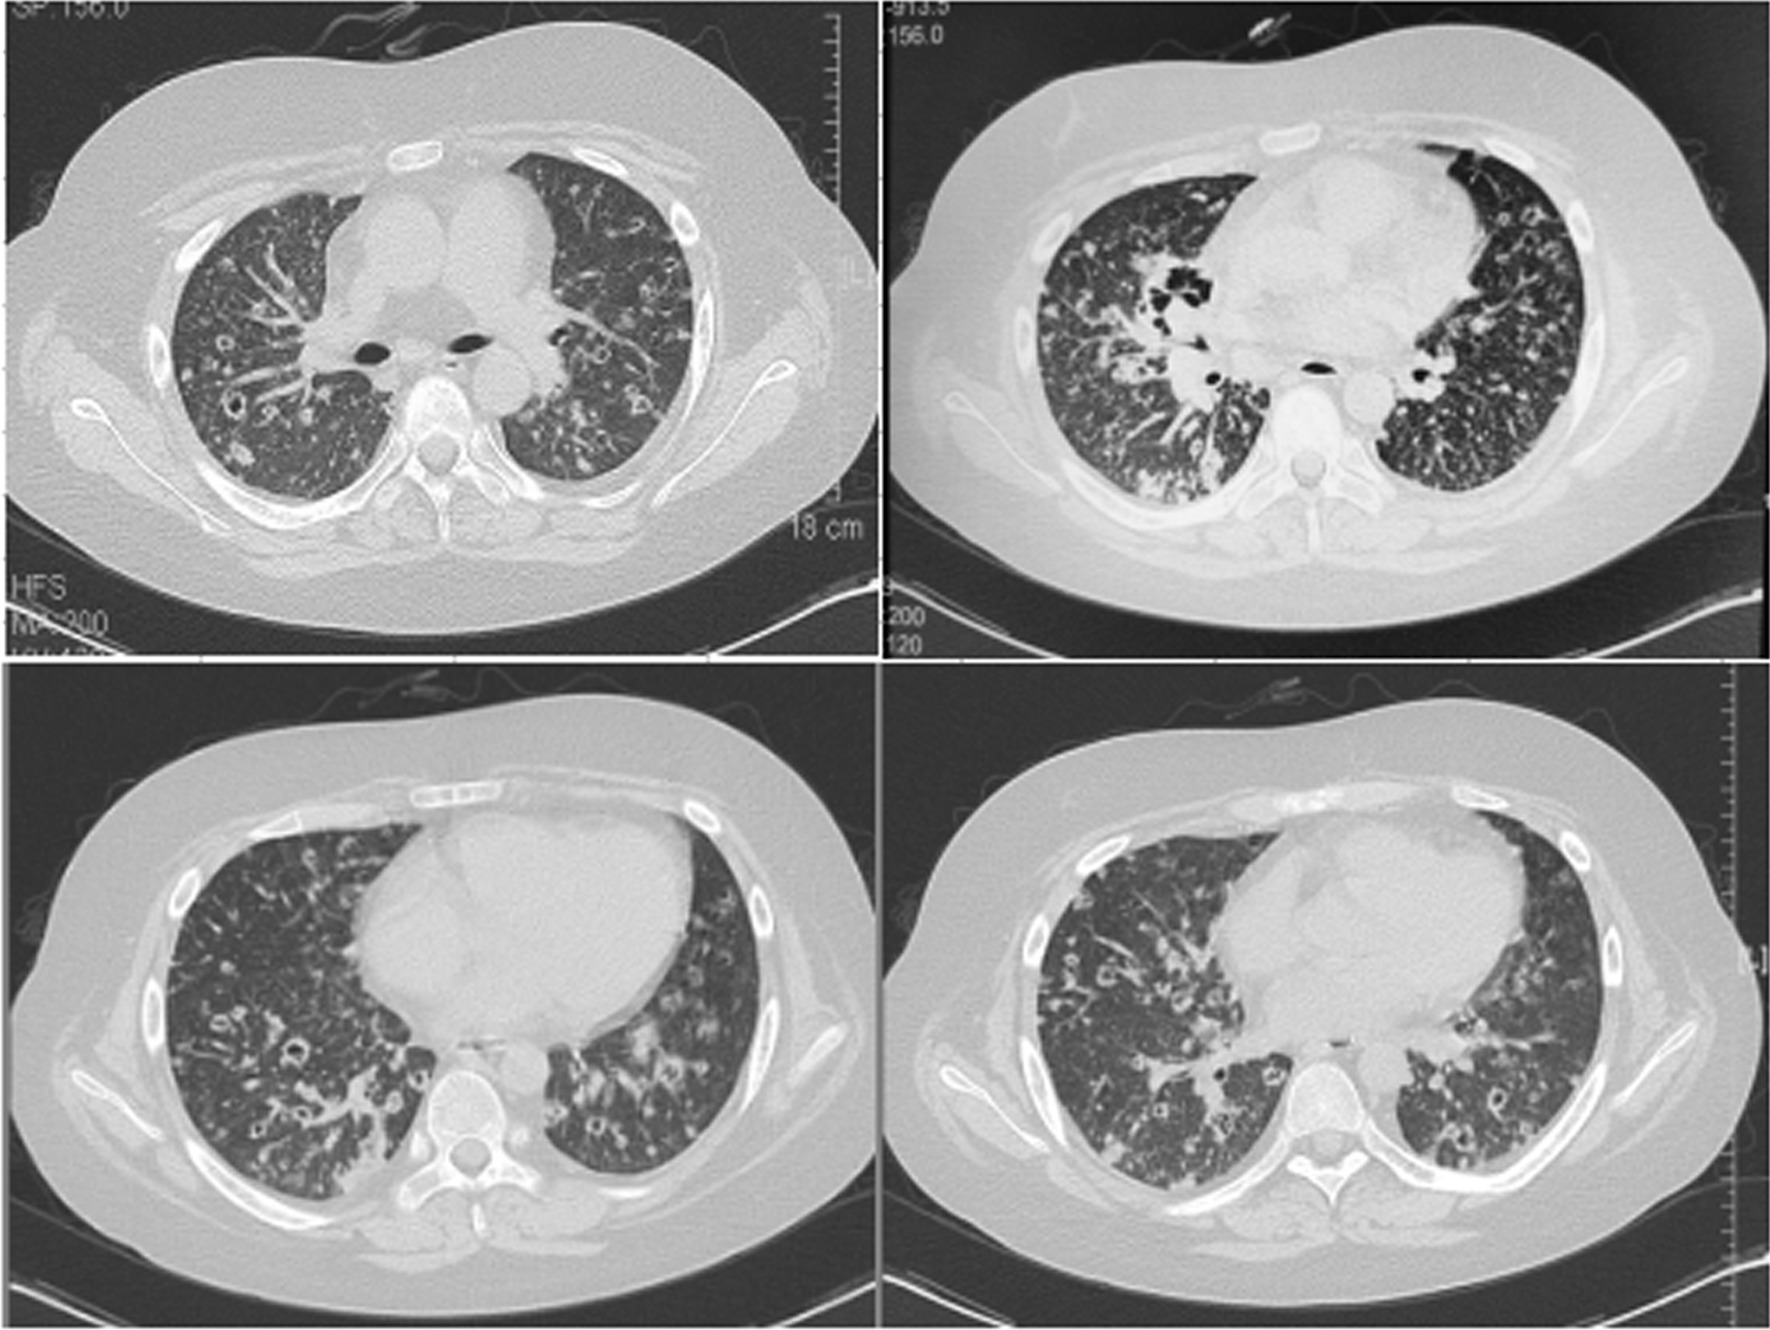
Chest computed tomography demonstrating a thick-walled cavitating lesion 2.5 cm × 3.5 cm in size in the right middle lobe with diffuse small nodules and cavities in both lungs
